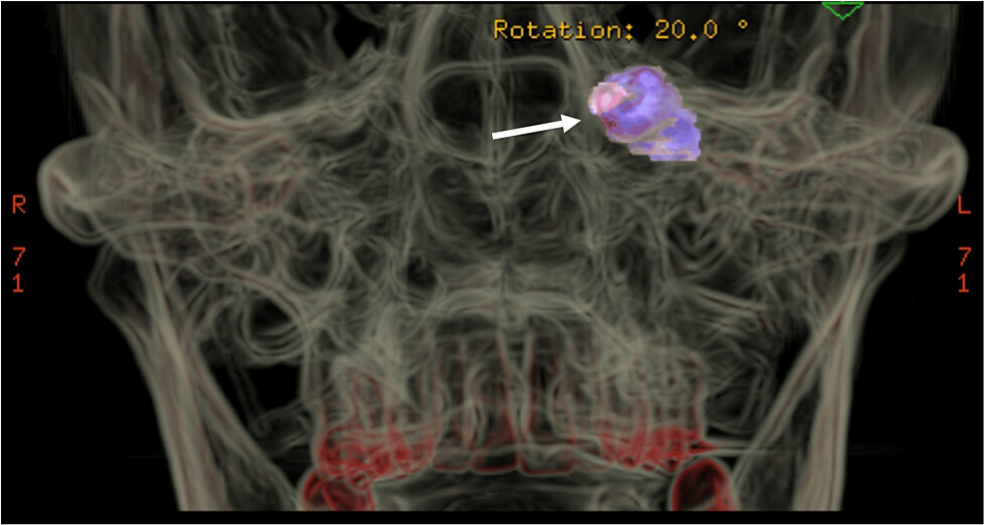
Three-dimensional reconstruction of preoperative maxillofacial CT scan Three-dimensional view of CT scan showing a highlighted ectopic tooth encroaching the posterosuperior wall of the left maxillary sinus
